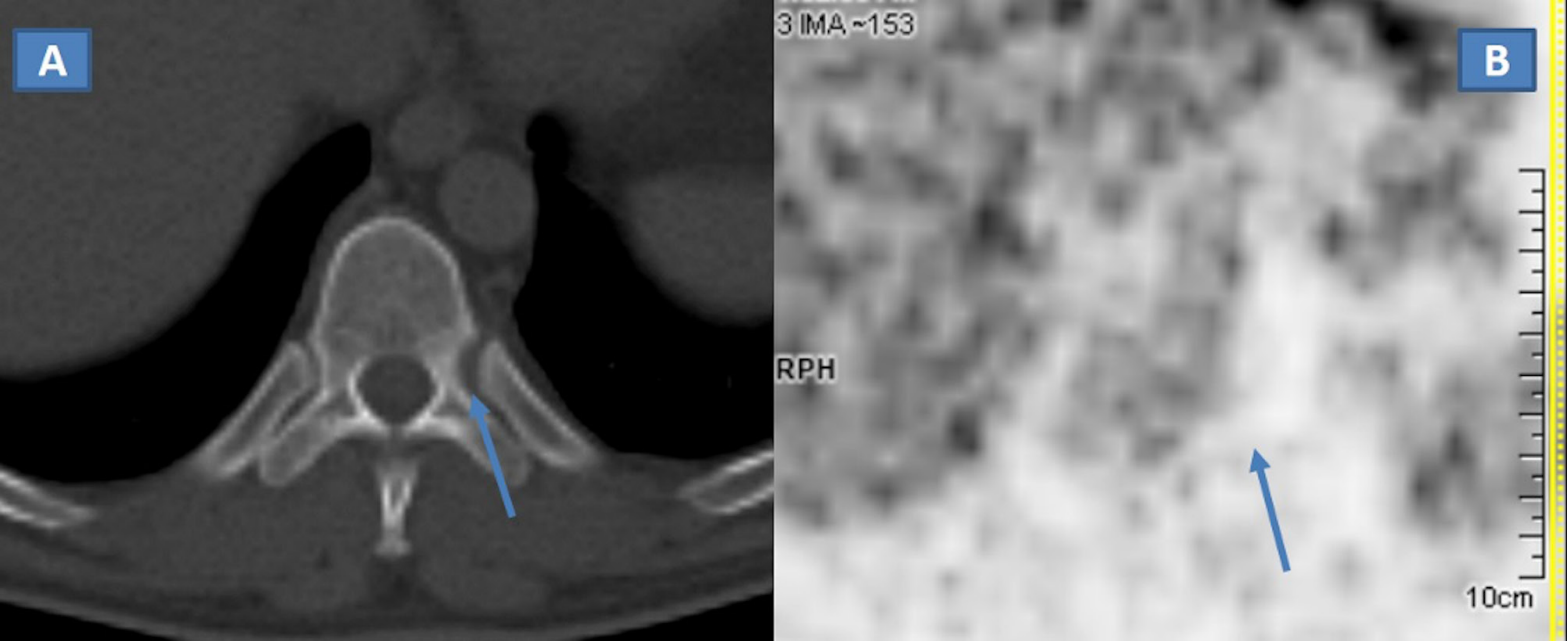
(A, B) Baseline CT scan revealed a widening of left T10 costovertebral
